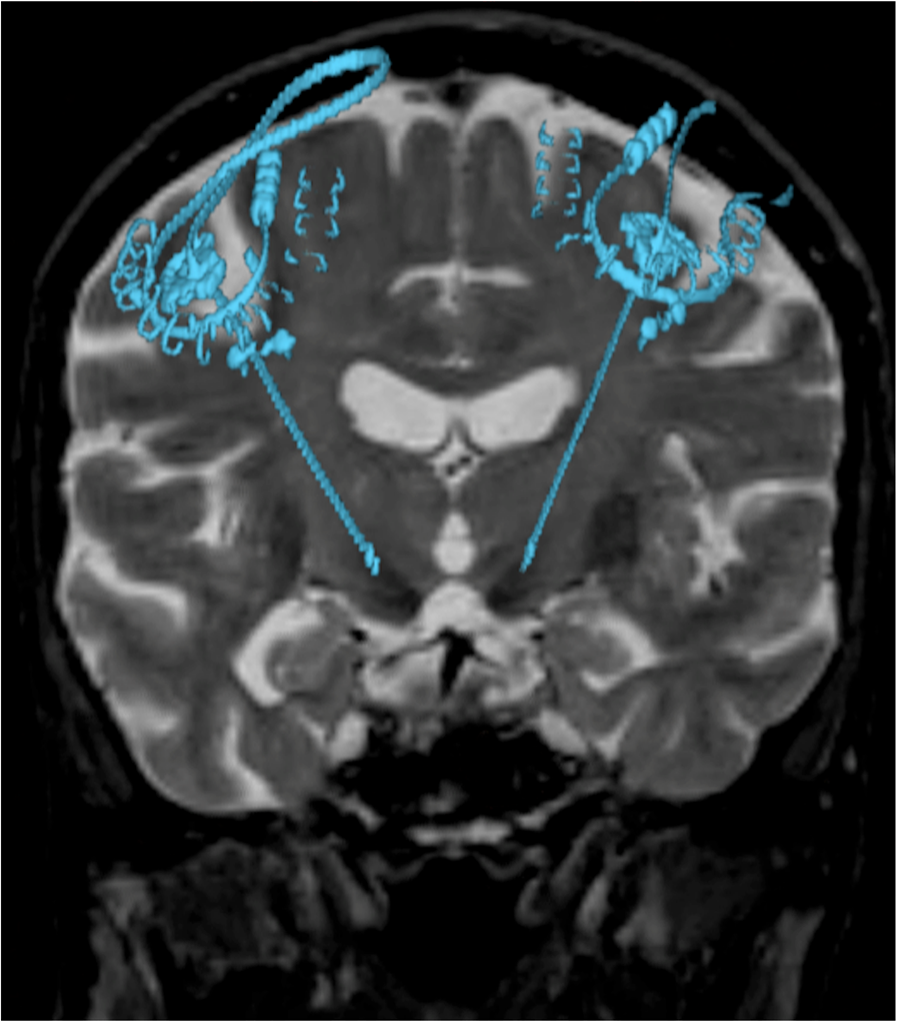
Coronal 3D T2WI fused with CT image shows electrodes of deep brain stimulation (DBS) in both subthalamic nuclei
